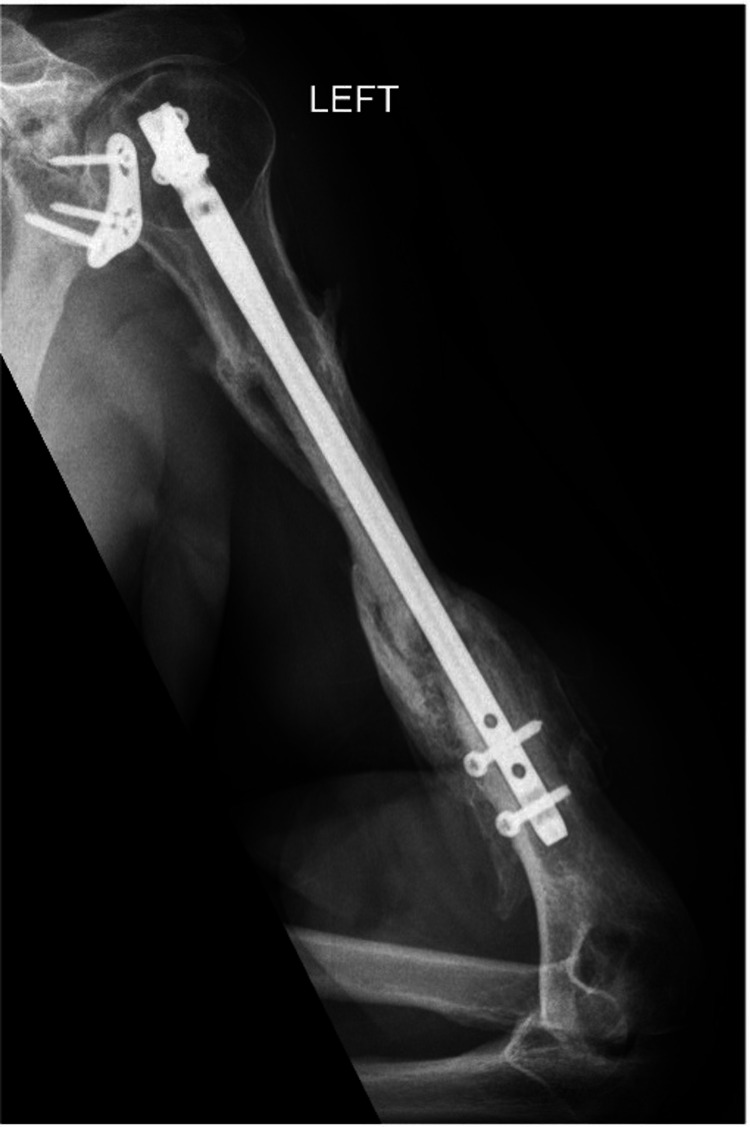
Lateral humerus radiograph demonstrating a healed comminuted fracture at one year follow up.
